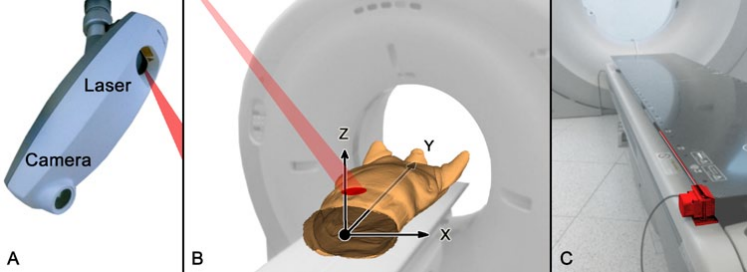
Installation and application of the Sentinel system: (a) Sentinel unit mounted at the ceiling; (b) patient setup and coordinate system; (c) the wire ruler (red) measures the couch position in Y direction and is mounted at the foot of the CT couch.
